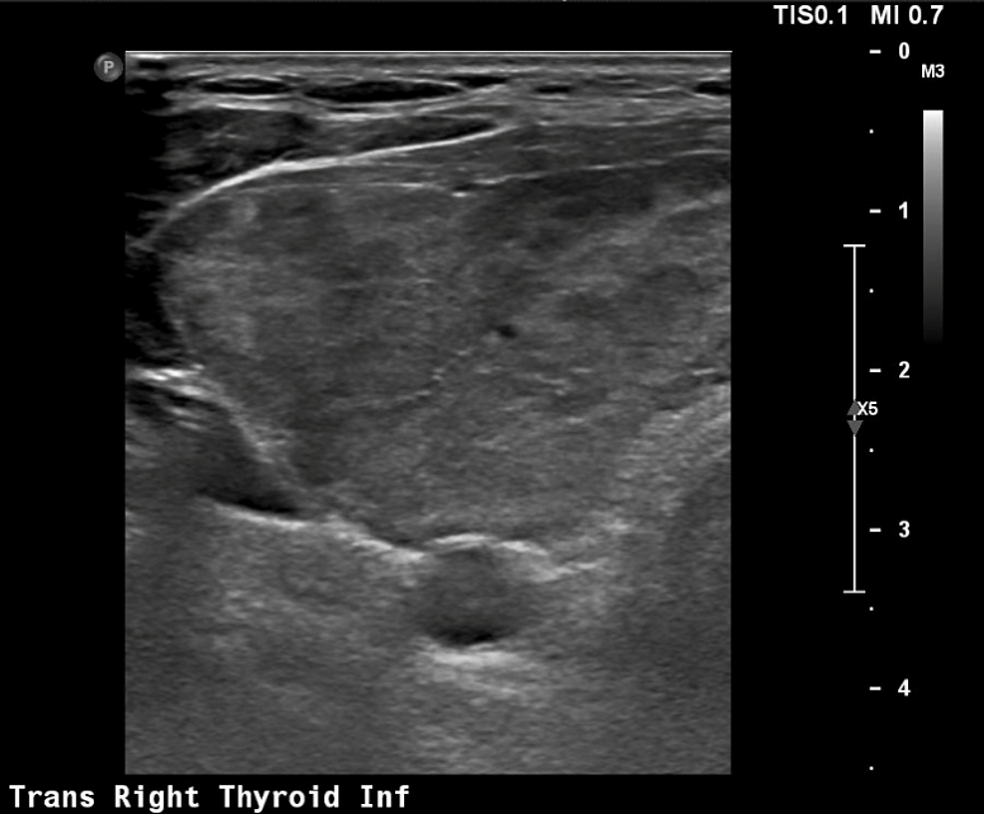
Thyroid ultrasound demonstrating significant heterogeneity and increased vascularity suggestive of thyroiditis.
